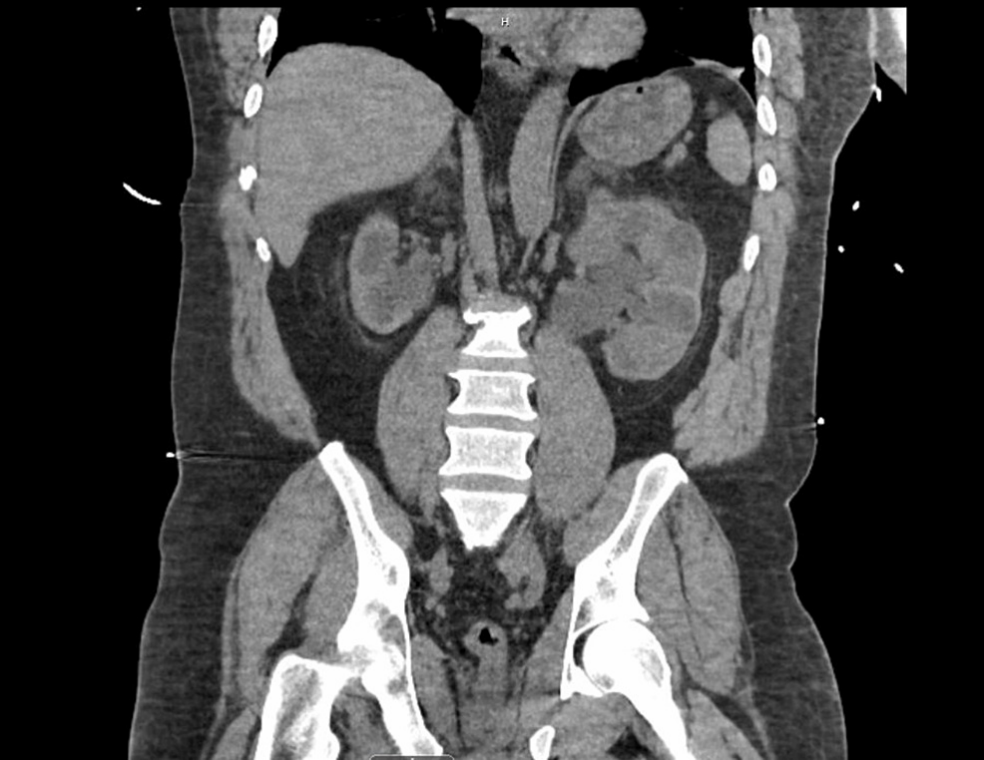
Coronal view on CT demonstrating severe left hydronephrosis with perinephric fat stranding.
